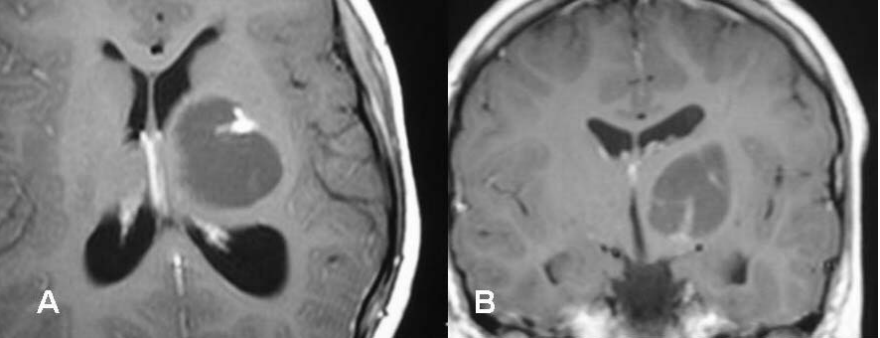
MRI brain showed left thalamic postoperative recurrent cystic pilocytic astrocytoma (solid and cystic part), before Gamma Knife surgery.
